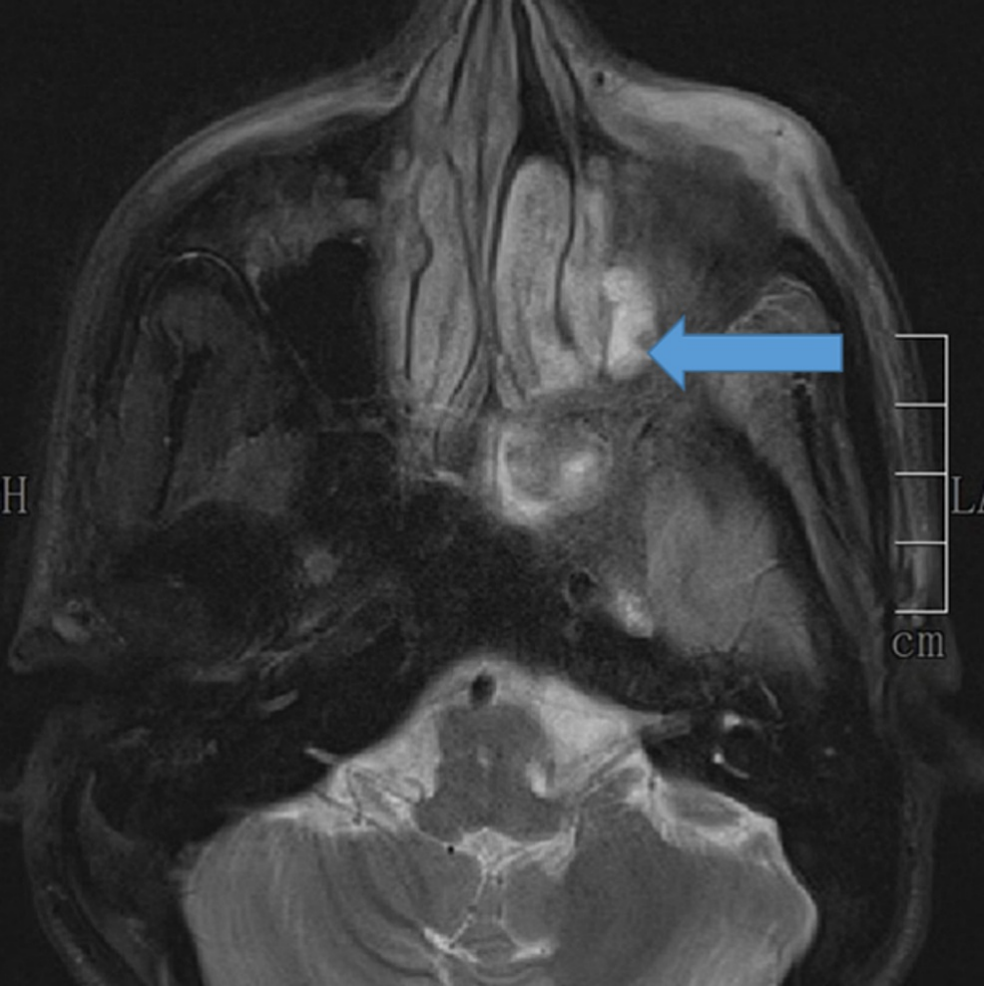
MRI- Fat suppression (FS) image showing extra-conal left orbital involvement
